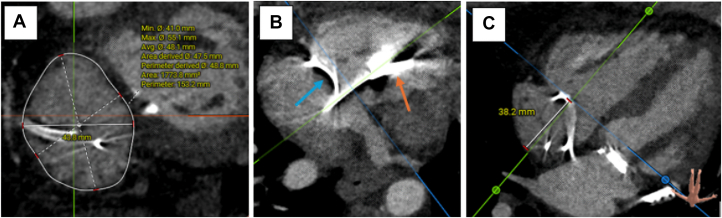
Screening Computed Tomography Assessment (A) Annulus sizing, (B) location of atrial lead (blue arrow) and ventricular lead (orange arrow), and (C) right atrial height measurement.
